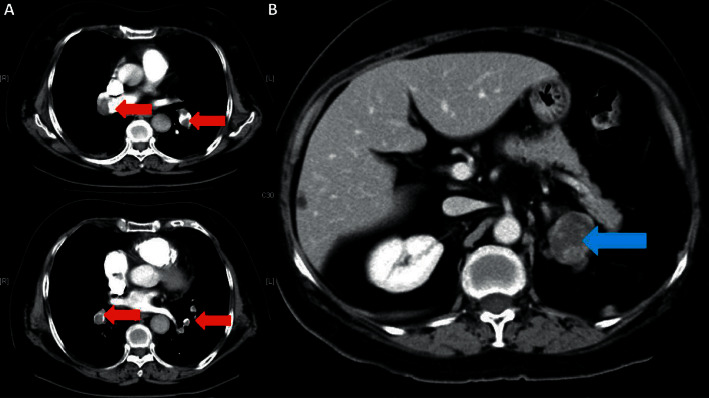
(a) CT pulmonary artery of Patient B on 25 August 2011 showing extensive bilateral pulmonary embolism (red arrow). (b) CT abdomen of Patient B on 13 October 2011. A 3.6 × 3.5 cm lobulated heterogeneously enhancing lesion with a focal calcified spot at the lateral limb of left adrenal with 30HU in pre-contrast scan (blue arrow).
